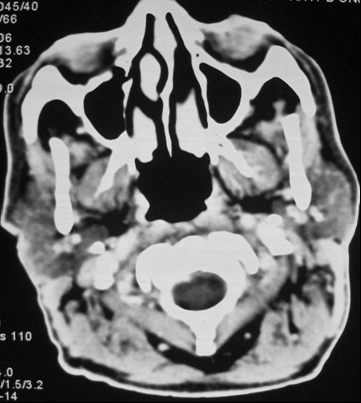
Computed tomography scan after chemotherapy. Complete regression of nasopharyngeal mass with cervical necrosis lymph nodes.
